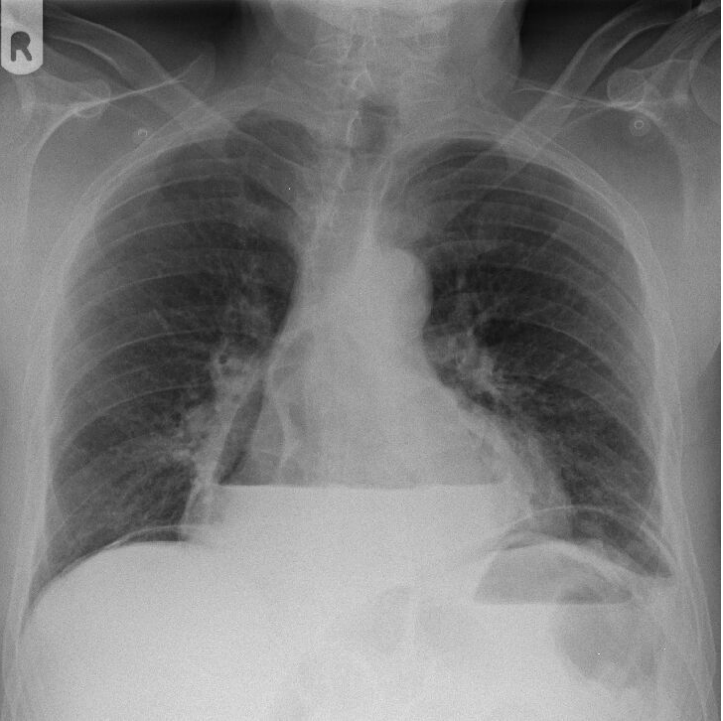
Chest x-ray at the emergency department. Posterior-anterior conventional radiograph of the chest with an intrathoracic air-fluid collection. Air-bubble in stomach. Free-intraperitoneal air inferior of both hemidiaphragms. Image suspect of stomach/bowel perforation and partial intrathoracic positioned stomach.
